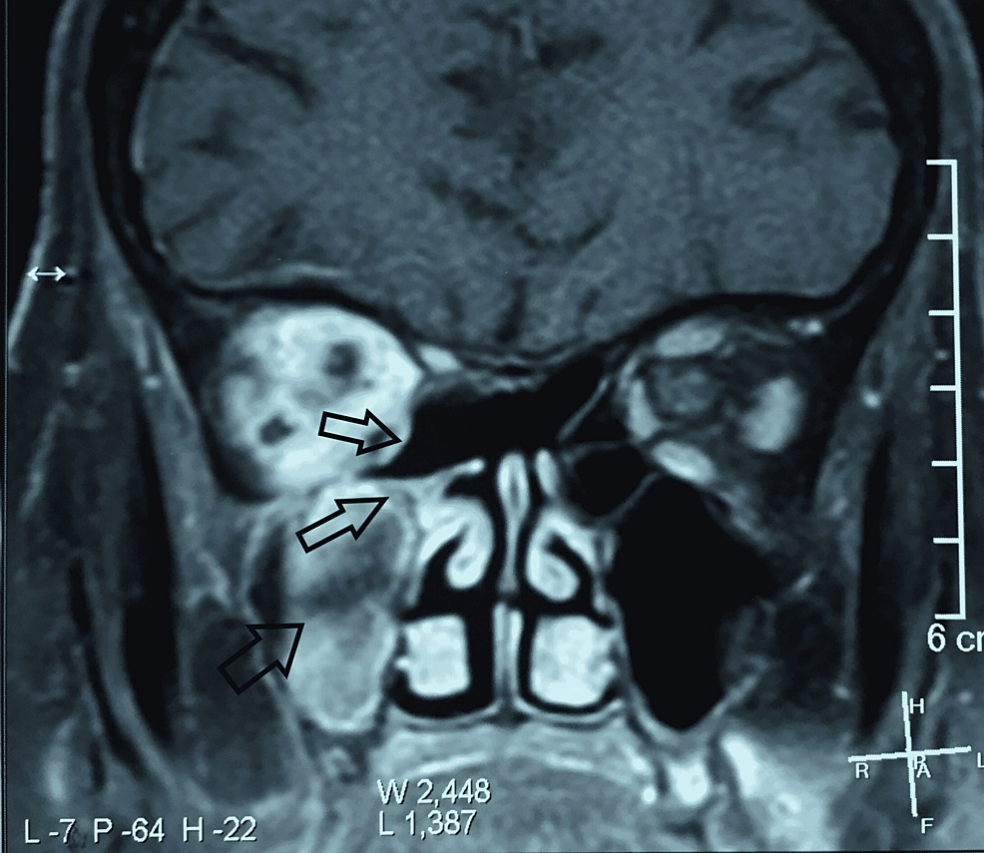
Magnetic resonance imaging of the sinuses showing right-sided frontal, maxillary and ethmoidal sinusitis
